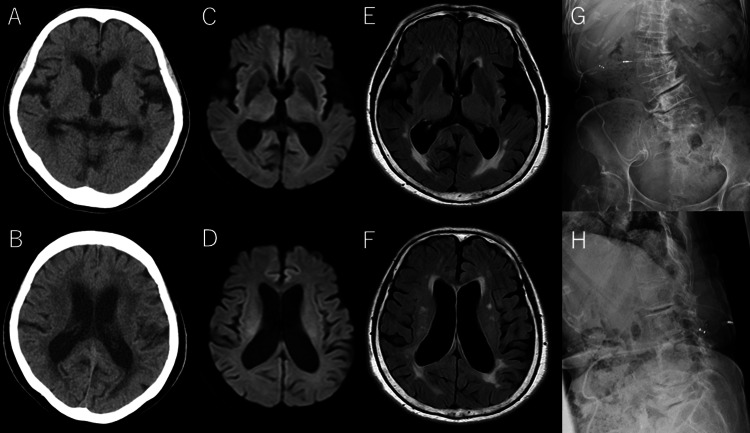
Follow-up imaging studies (A, B) Head CT, (C, D) MRI diffusion-weighted images, and (E, F) MRI FLAIR images show no evidence of new lesions. (G, H) Abdominal X-rays in frontal and lateral views demonstrate no catheter disconnection or migration. FLAIR: fluid-attenuated inversion recovery
